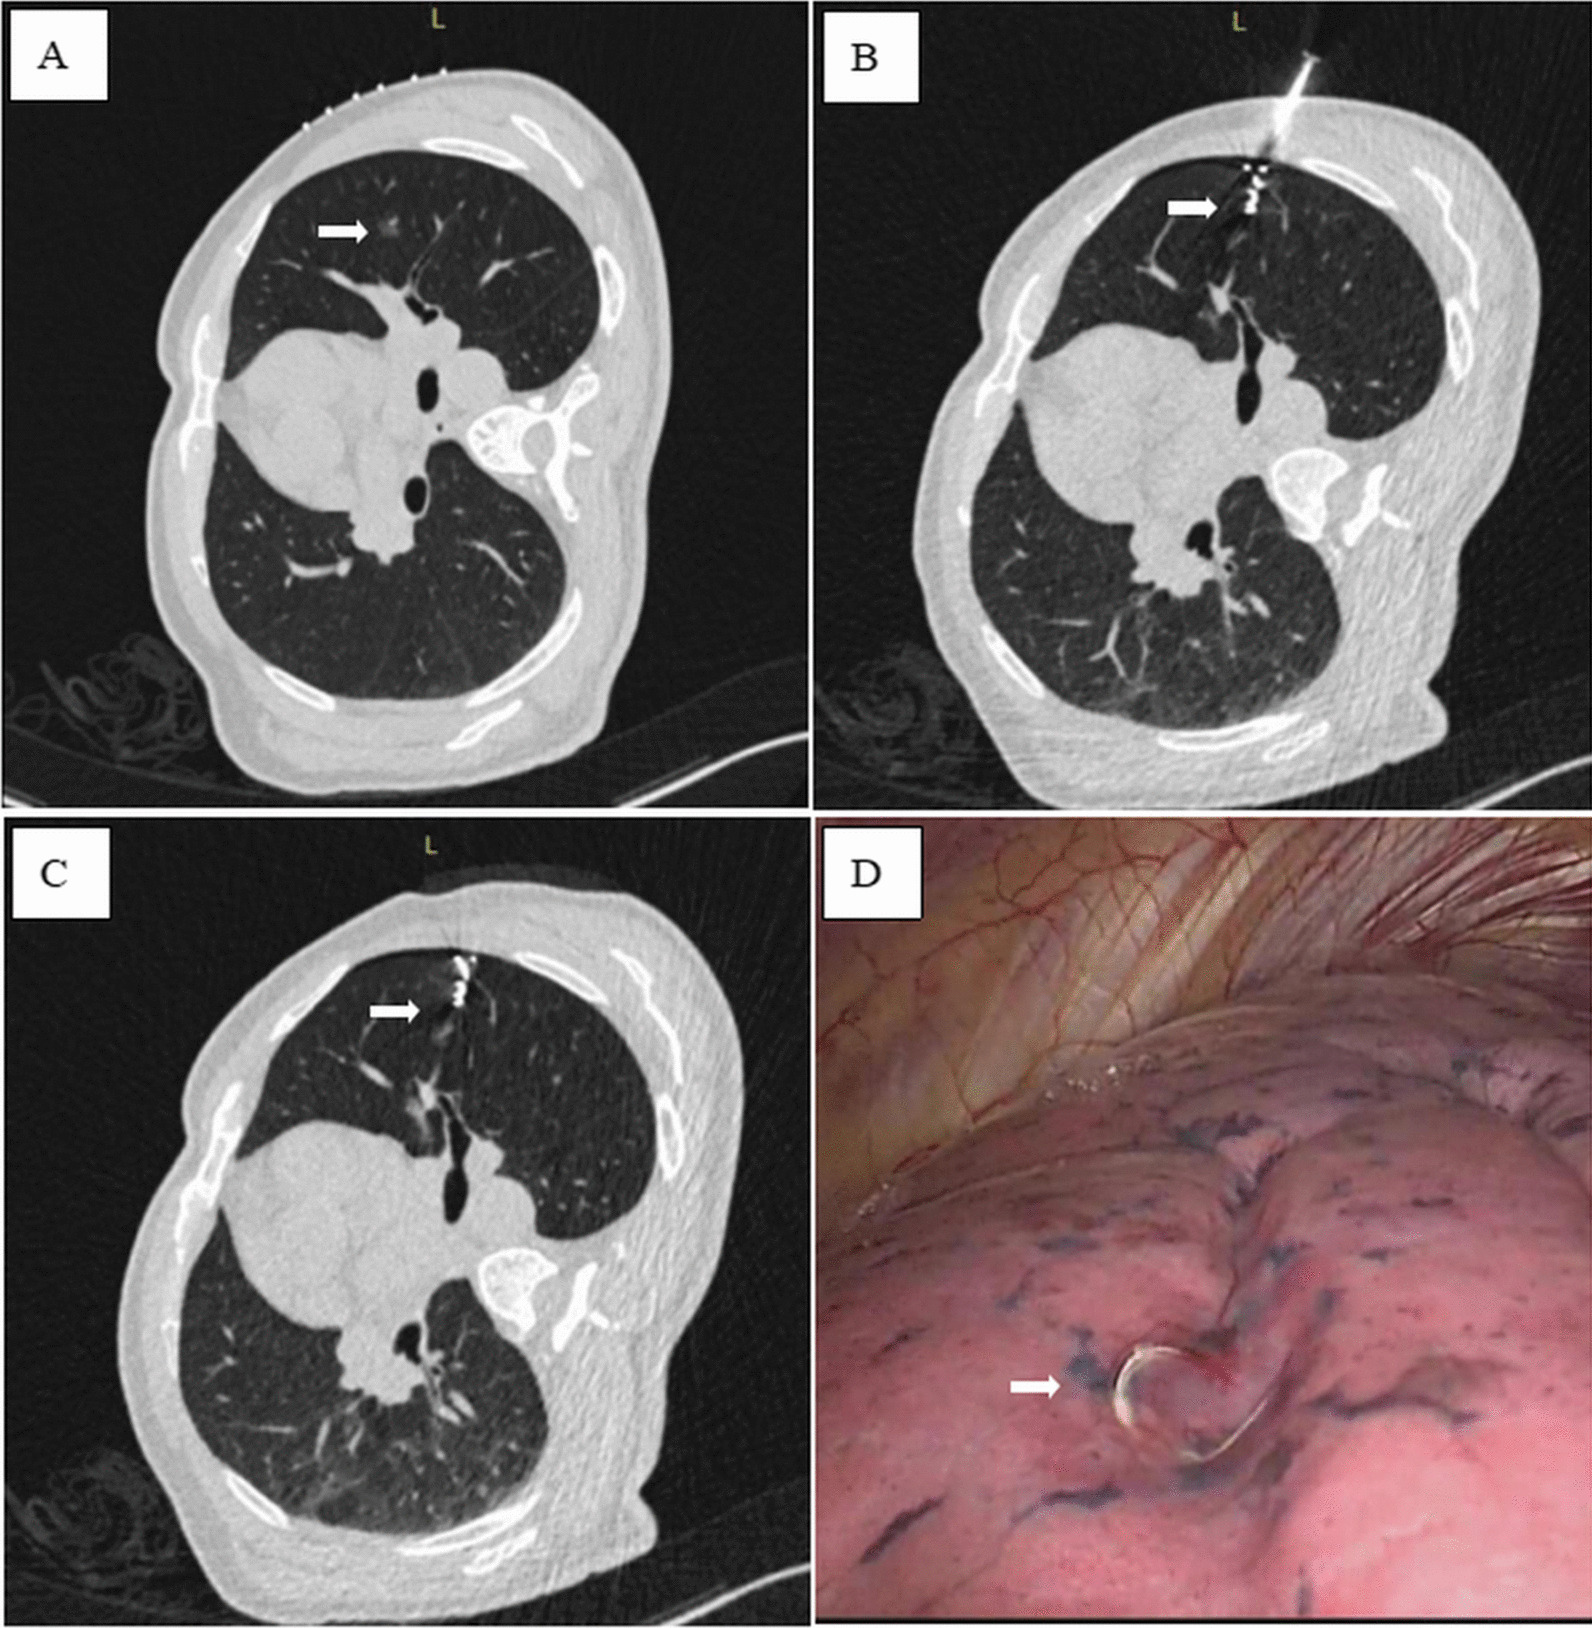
Computed tomography (CT)-guided micro-coil localization on a 48-year-old male with adenocarcinoma in situ in the right upper lobe. A CT image showing the lesion (arrow) in the right upper lung lobe; B CT image showing introducer needle was inserted into the lung and positioned next to the lesion (arrow); C The microcoil was released with the superficial end of the microcoil beyond the visceral pleura and the deep end coiled in the lung parenchyma adjacent to the lesion (arrow). D Superficial end of the microcoil (arrow) was visualized during video-assisted thoracoscopic surgery
